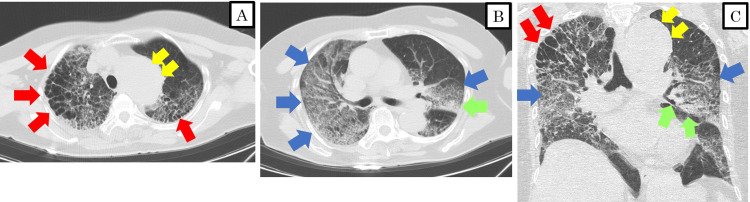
Chest CT images on admission (A, B) Axial view of chest CT imaging; (C) Coronal view of chest CT imaging CT: computed tomography
